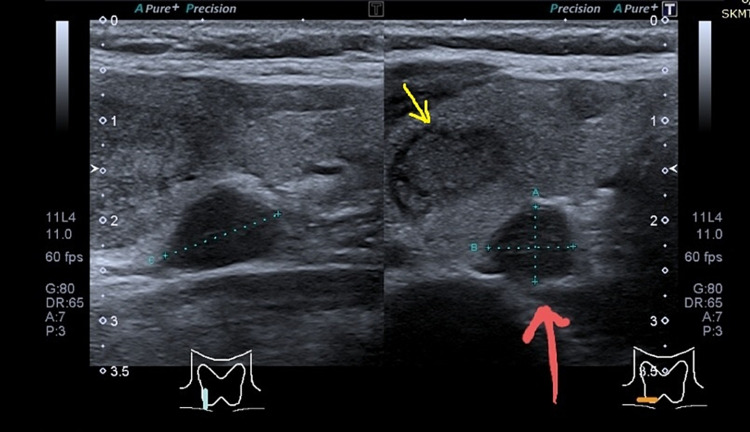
Ultrasound of the neck showing right thyroid lobe nodule (arrow in yellow) and right parathyroid gland (arrow in red)
